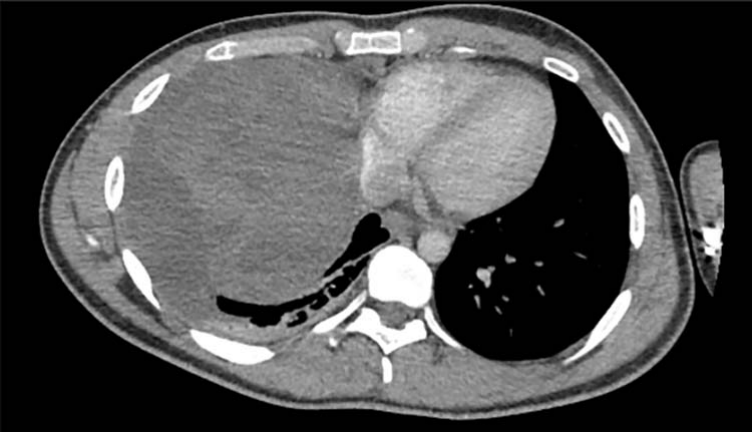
Axial view of CT of thorax with intravenous contrast demonstrating large enhancing and heterogenous mass of the right hemithorax; right-sided pleural effusion is also seen.
